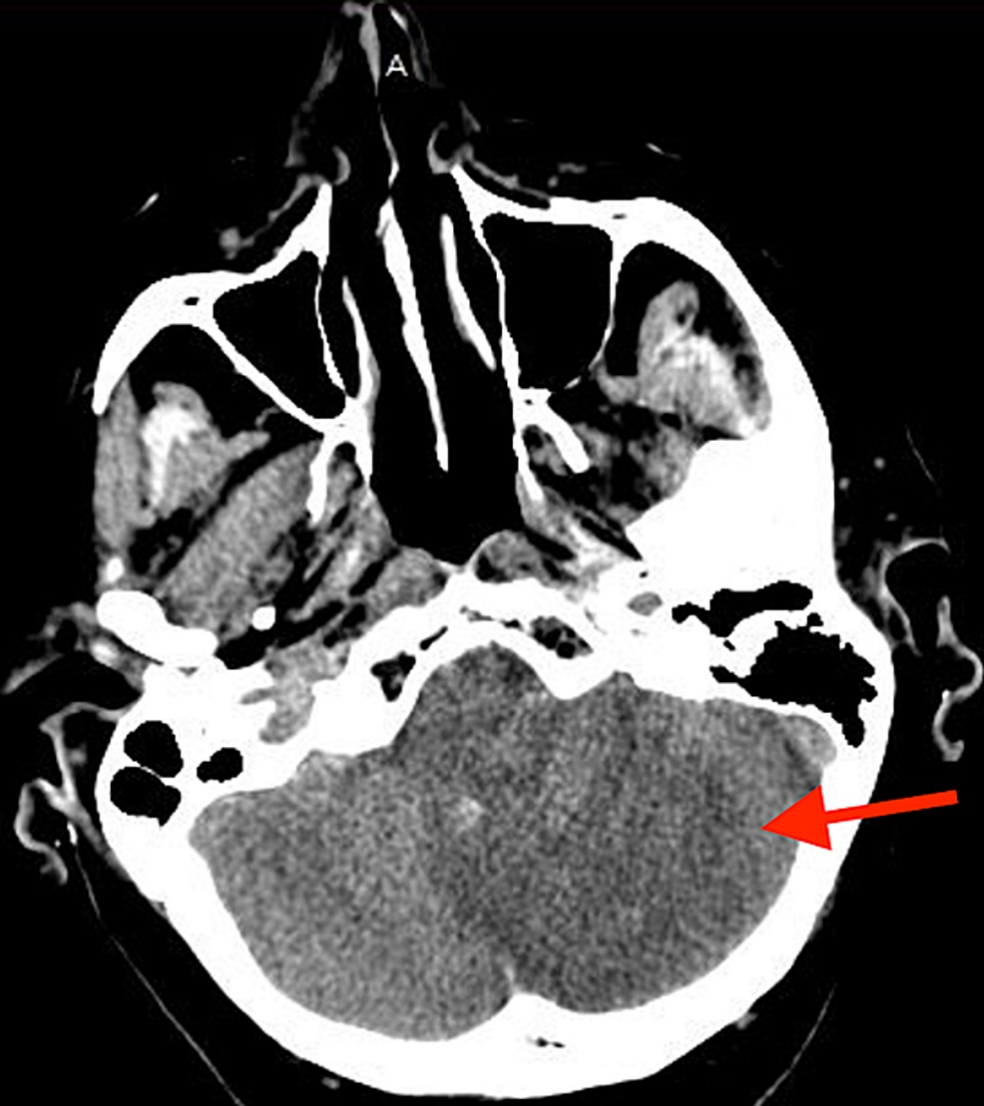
Computed tomography scan of the head without contrast showing acute infarct of the left cerebellar hemisphere with the hemorrhagic conversion with effacement of the fourth ventricle.
